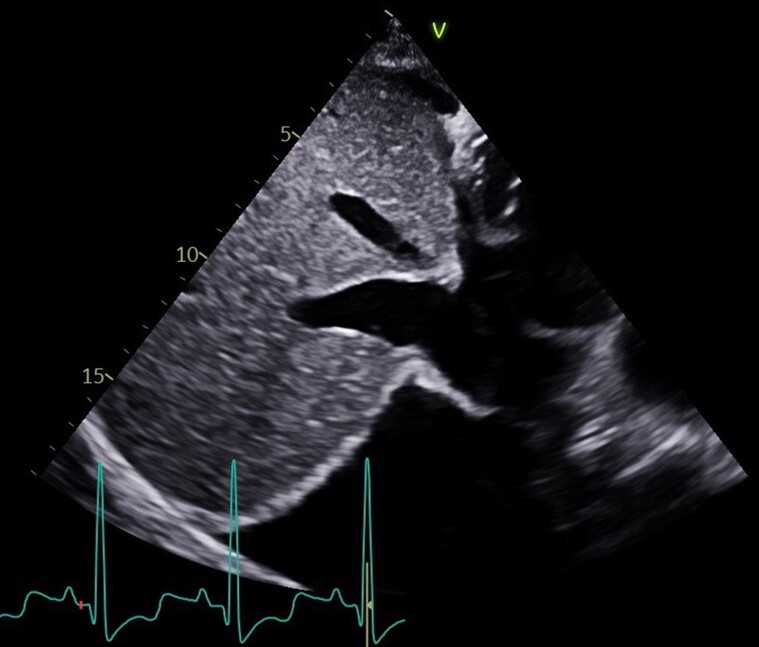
Two-dimensional diaphragm ultrasound showing the diaphragm region from the subcostal view used for two-dimensional strain imaging analysis. The bright line surrounding the liver indicates the diaphragm.
